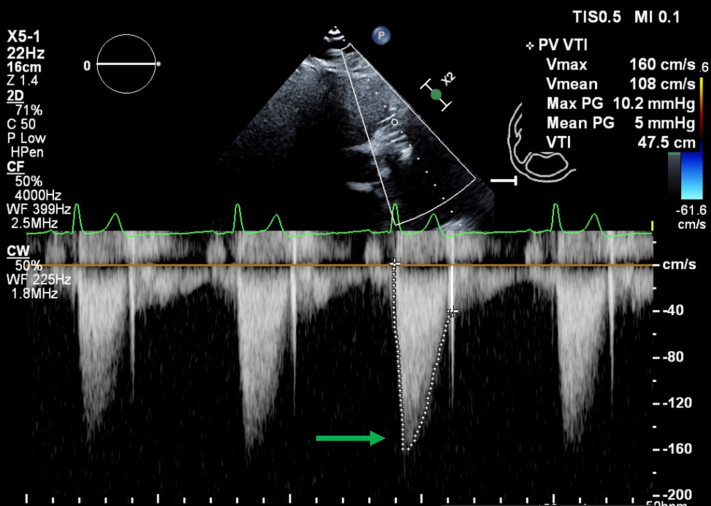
Transthoracic Echocardiogram After 4 Weeks of Anticoagulation Imaging demonstrates a continuous-wave (CW) Doppler measurement of the mean gradient, indicated by the green arrow, of 5 mm Hg. Abbreviations as in Figure 2.
